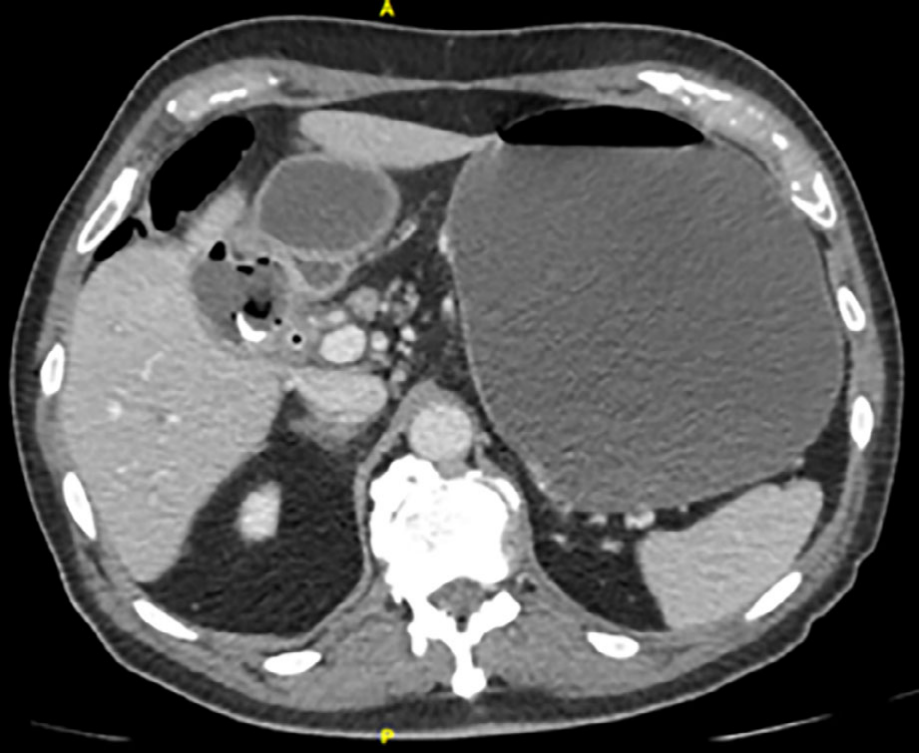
Axial image from abdominal computed tomography shows a calcified structure in the duodenal bulb and distended stomach.
